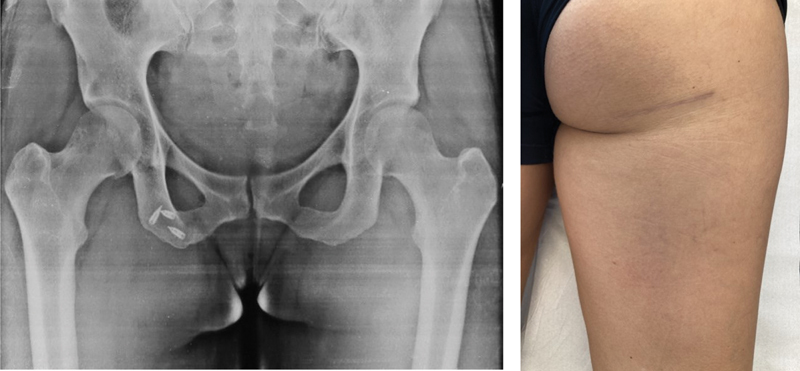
Final radiographic appearance with anchors in the tendon footprint; scar 3 months postoperatively.
